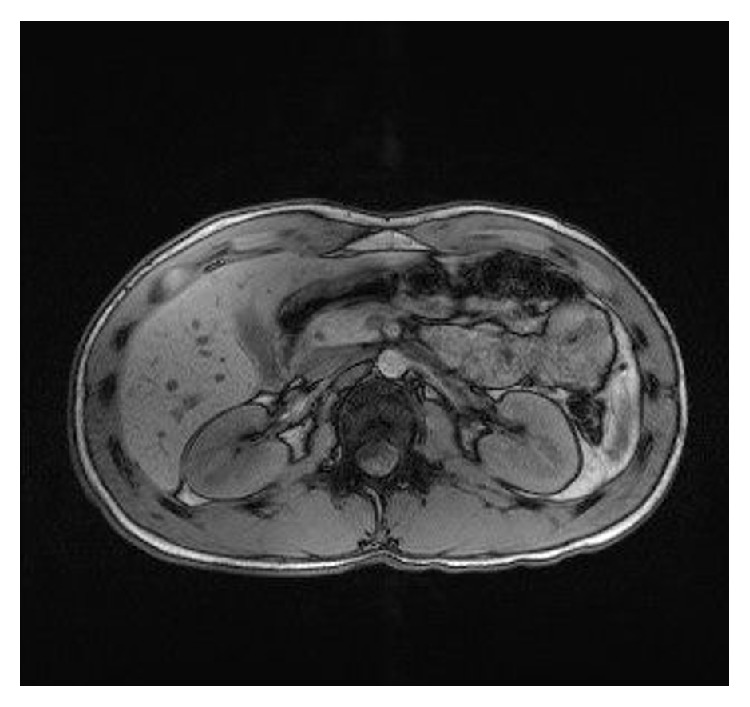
Follow-up MRI with fluid-filled hepatic lesions.
